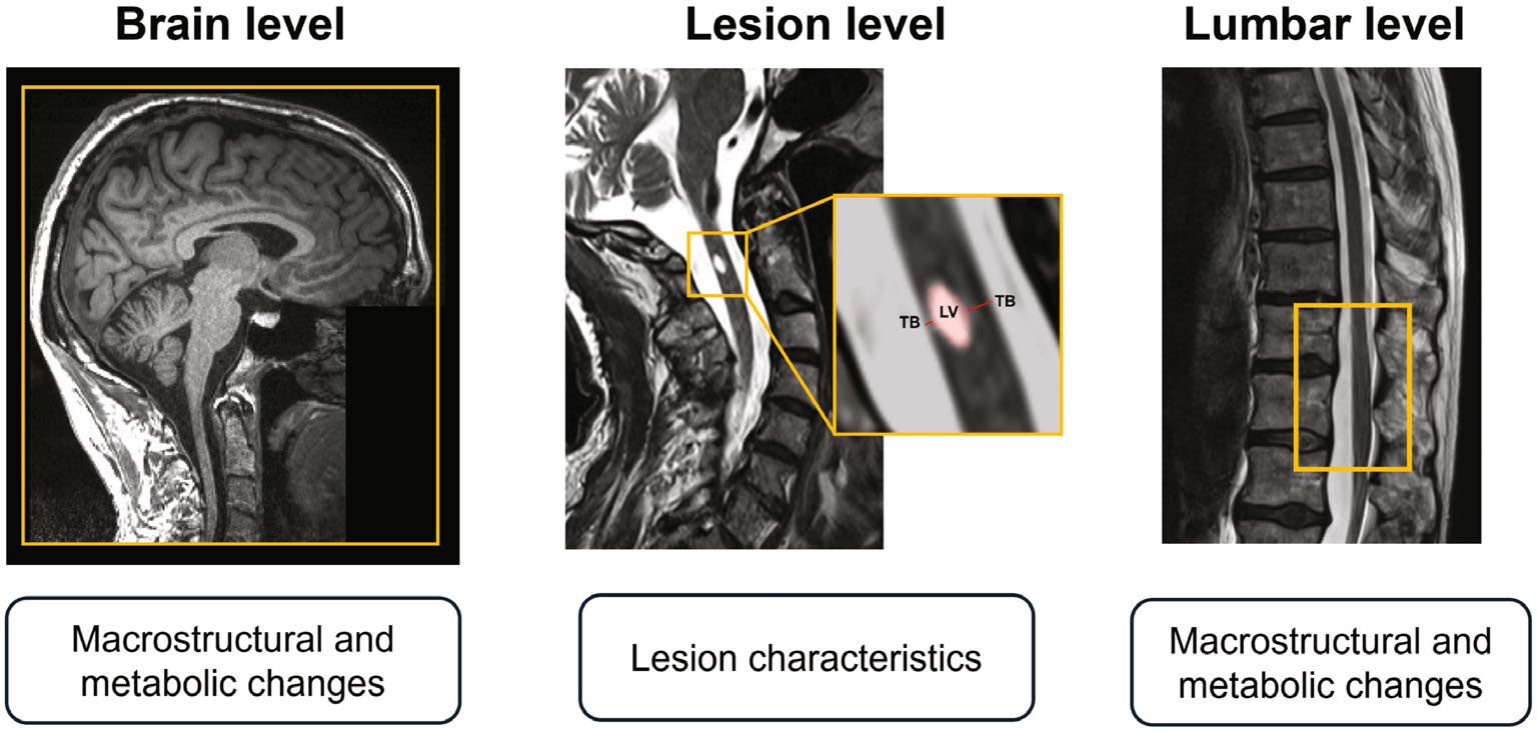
MR acquisition across the motor system. Example of images acquired on an SCI patient across the motor system, including a 3D T1‐weighted acquisition of the brain, a sagittal T2‐weighted MRI of the lesion level with representation of the lesion parameters (lesion volume (LV) and tissue bridges (TB)), and a sagittal T2‐weighted MRI of the lumbar level, with the lumbar cord enlargement highlighted.
